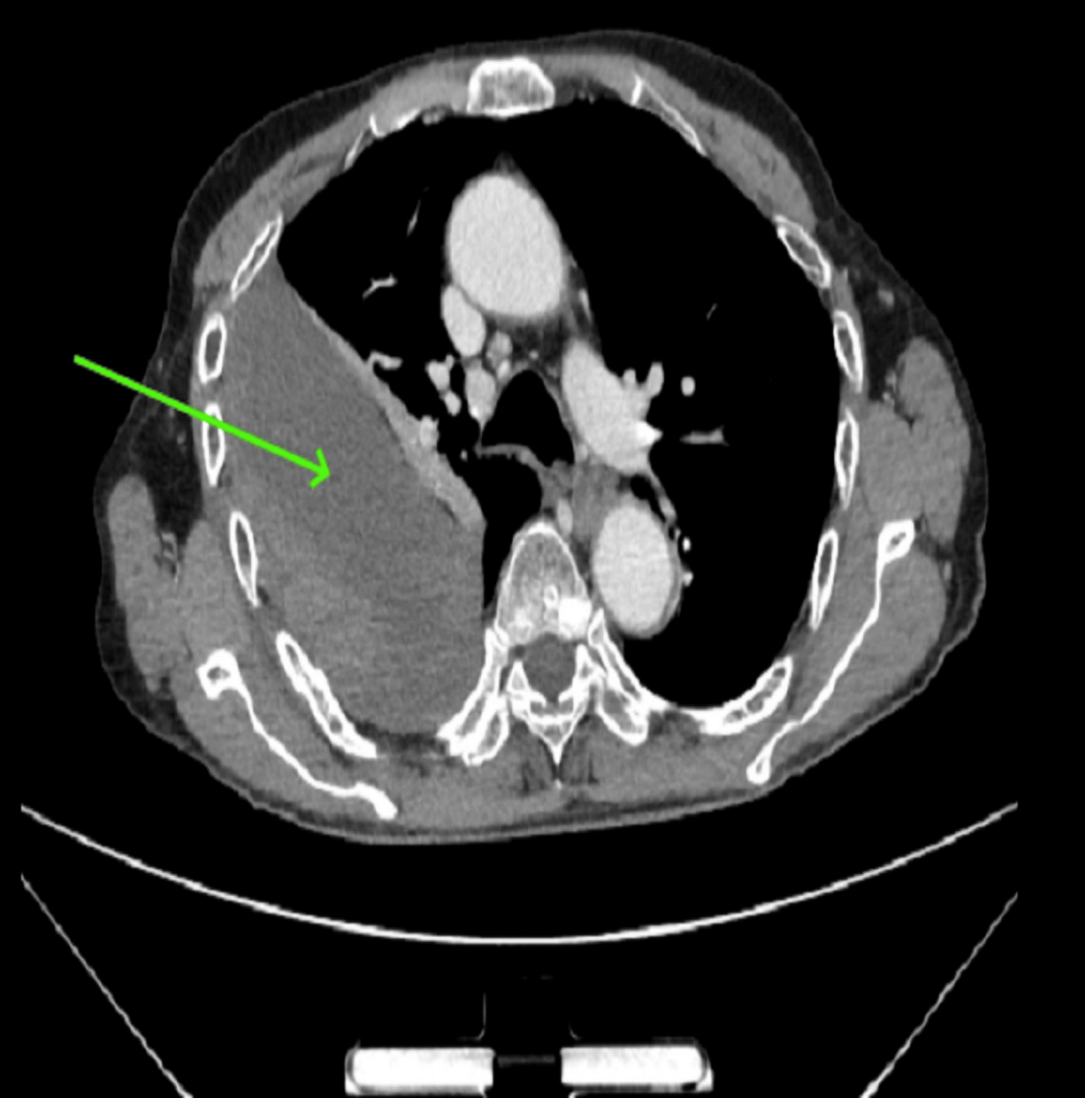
Axial section contrast-enhanced CT of the chest showing a well-defined heterodense with hyperdense extrapleural collection on the right side (green arrow) CT: computed tomography
